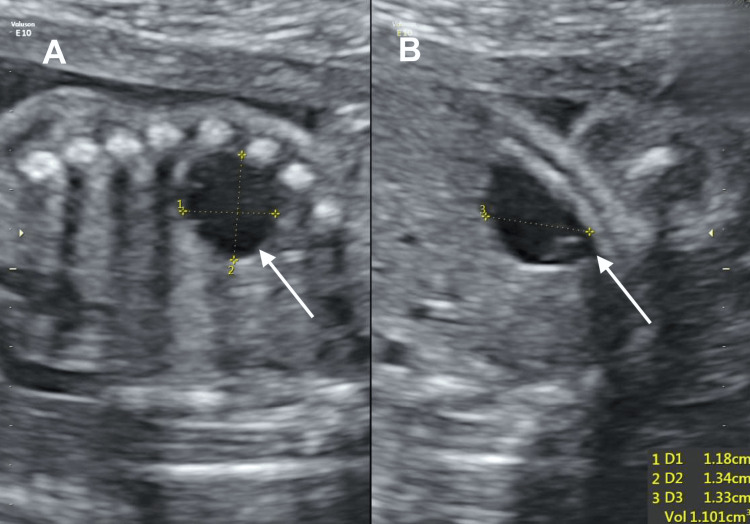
Ultrasonography at 23+4 weeks showing an avascular hepatic cyst The arrows point to the fetal hepatic cyst seen on ultrasonography. A) Ultrasound showing the presence of an avascular hepatic cyst measuring 1.18 cm x 1.34 cm (D1 = depth, D2 = width) B) Ultrasound showing the presence of an avascular hepatic cyst measuring 1.33 cm (D3 = length)
